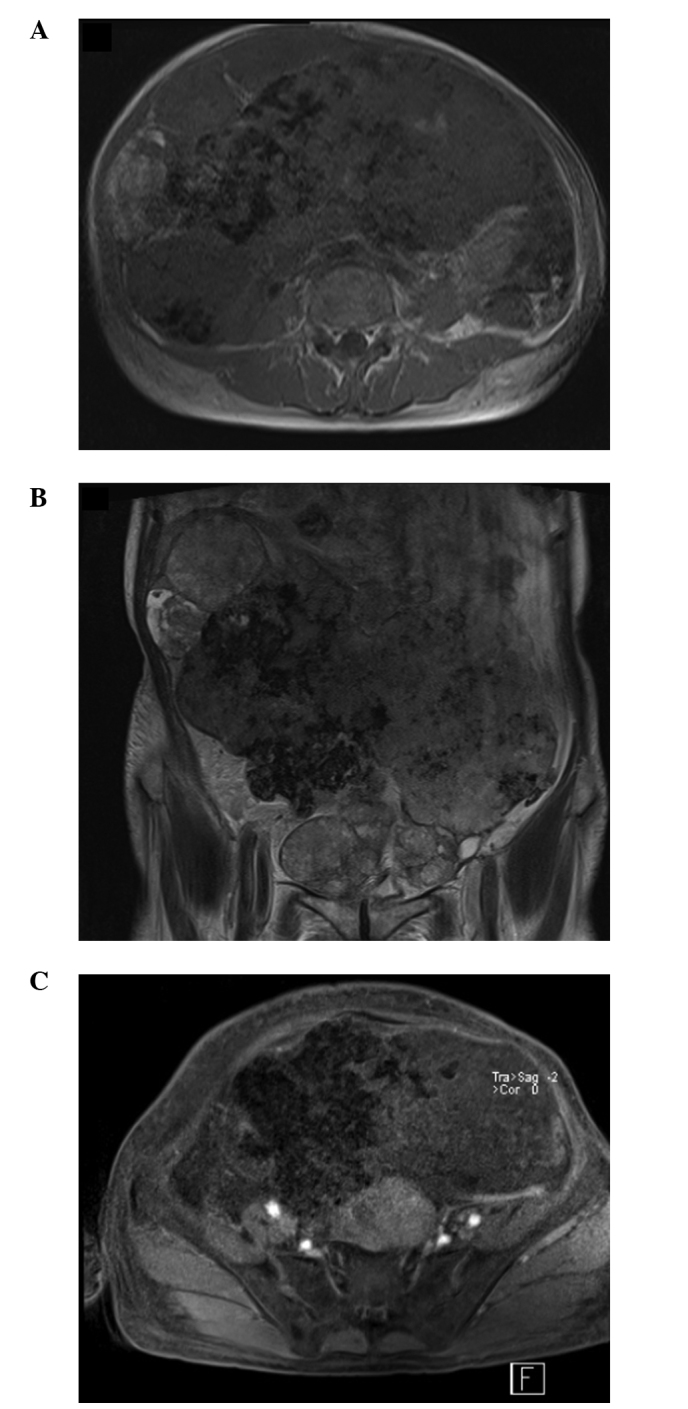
(A) Unenhanced transverse T1-weighted images reveal low signal intensity of the lesions, whereas the central area exhibits an even lower intensity. (B) Unenhanced coronal T2-weighted images predominantly reveal marginally high signal intensities of the lesions, whereas the central area exhibits low intensity. (C) Enhanced magnetic resonance imaging reveals peripheral and mild speculated enhancement.
